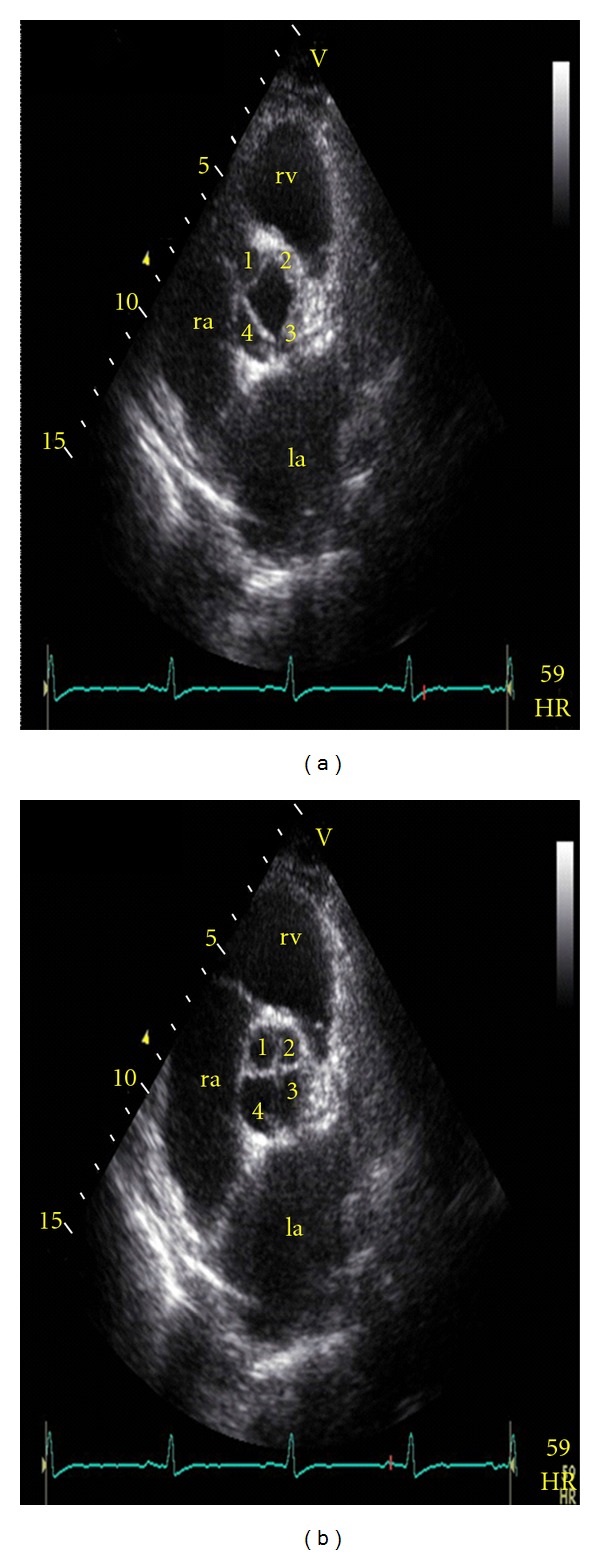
Transthoracic echocardiography images. Short axis view during systole (a) and diastole (b). ra: right atrium, la: left atrium, and rv: right ventricule.
